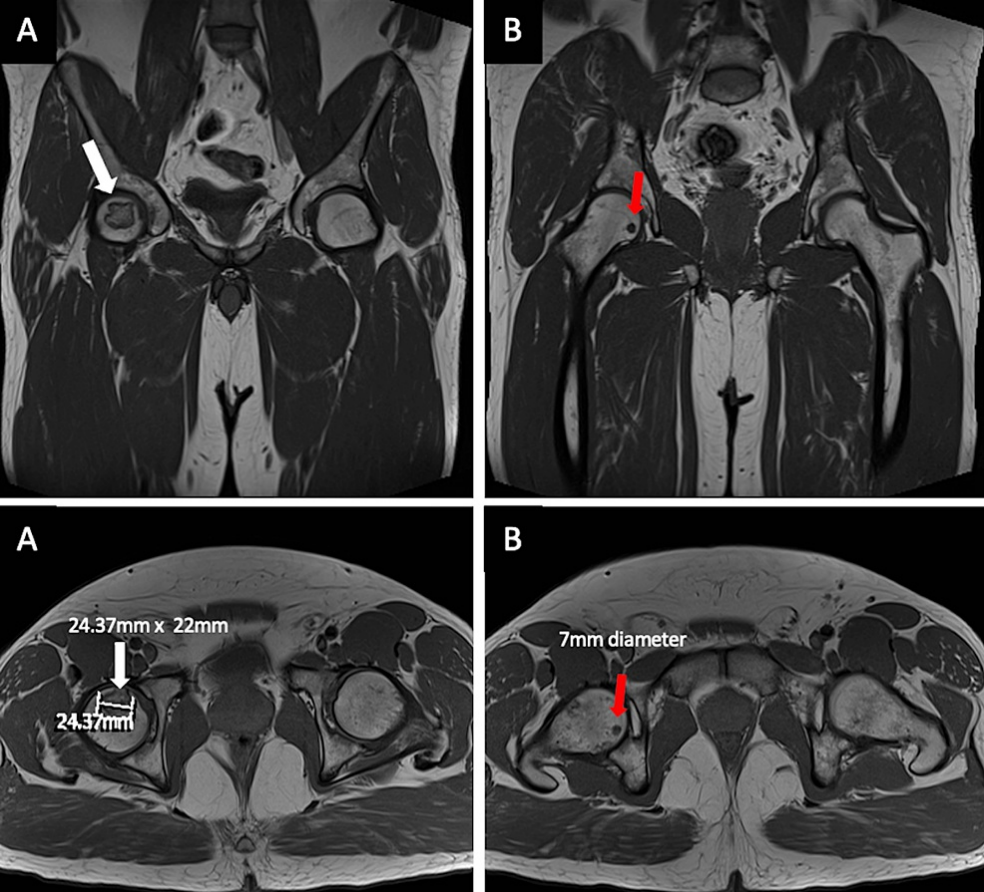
MRI radiographs demonstrating (A) a larger AVN lesion (white arrows), abutting the right anterior superior femoral cortex, measuring 25 x 22 mm, and (B) a smaller AVN lesion (red arrows), located more medially, measuring 7 mm in diameter. The right femoral head remains spherical in shape, with no articular irregularity, collapse, or surrounding tissue degeneration. AVN: avascular necrosis.
